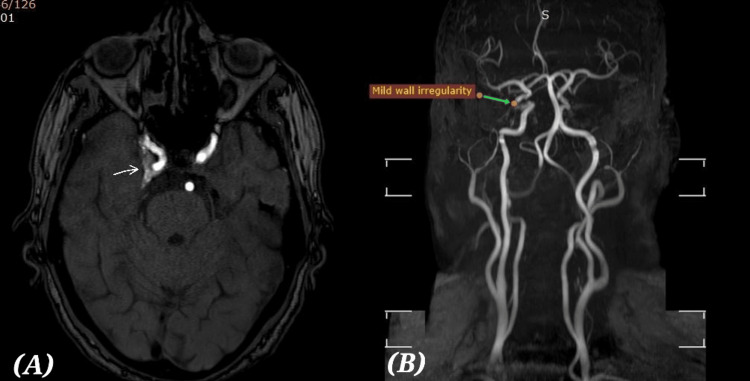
MRI + Angiogram (A) MRI (axial time of flight): Asymmetric T2 intermediate signal intensity enhancing soft tissue in the region of right cavernous sinus causing bulging of the right lateral margin and partial obliteration of the superior aspect of right Meckel's cave suspicious of Tolosa-Hunt (B) MRA: Mild wall irregularities of the right internal carotid artery (ICA) cavernous segment likely due to the MRI findings
